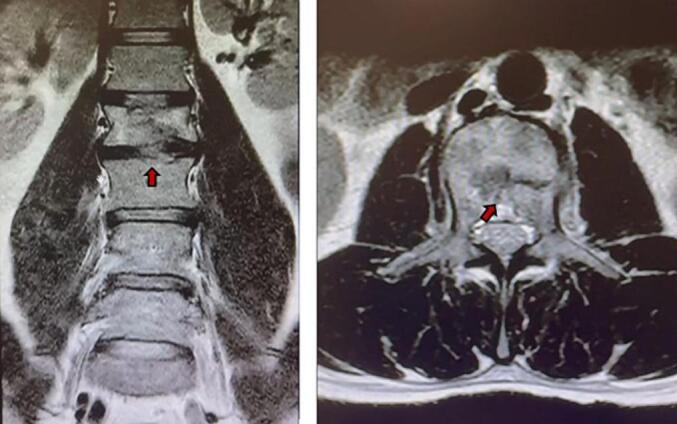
Magnetic resonance imaging (MRI) at final follow-up (5 months and 40 days after the trauma) demonstrating fracture healing (coronal and transverse views).
